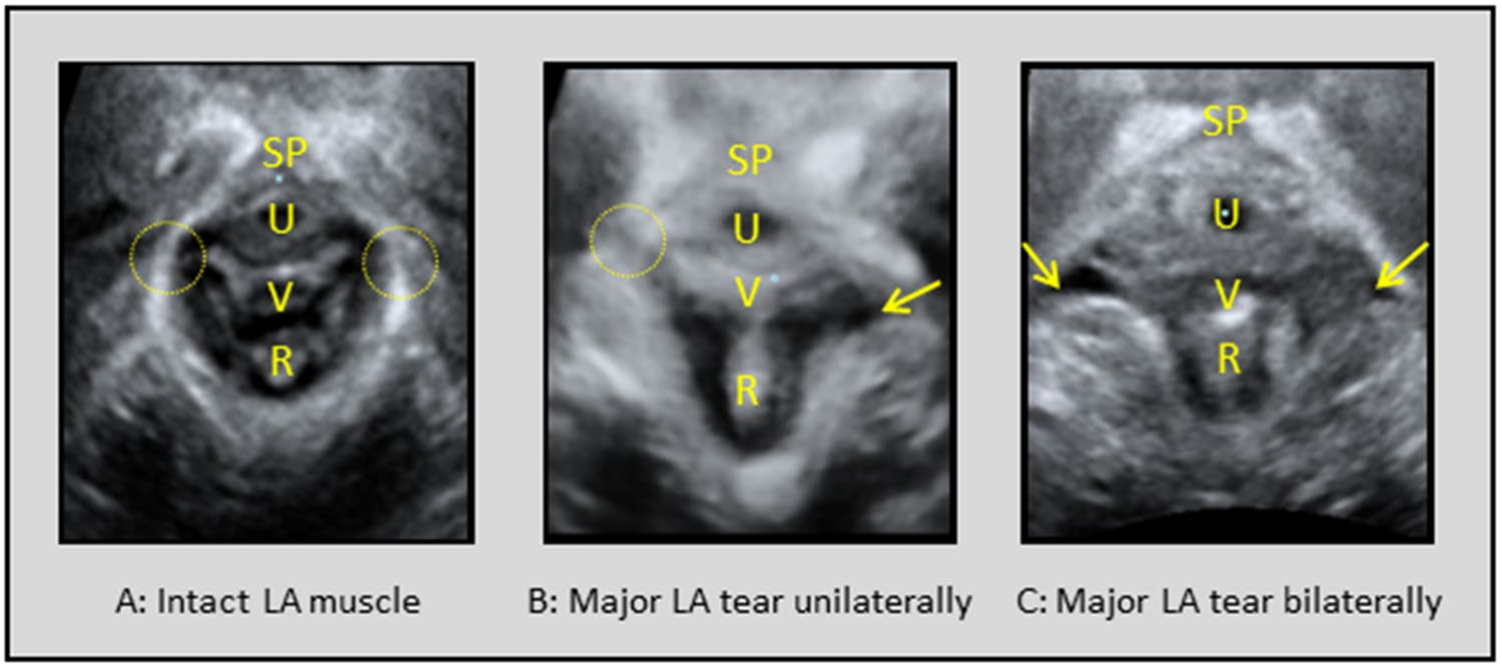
Tomographic ultrasound imaging of the levator ani (*LA*) muscle after vaginal delivery in the axial plane of minimal hiatal dimensions in render mode [19]. Normal muscle insertion of the LA muscle is marked by the *dotted circle*. Abnormal LA muscle insertion is marked by an arrow. Image A shows an intact LA muscle, B shows a major LA tear unilaterally, and C shows a major LA tear bilaterally. *SP* symphysis pubis, *U* urethra, *V* vagina, *R* rectum
